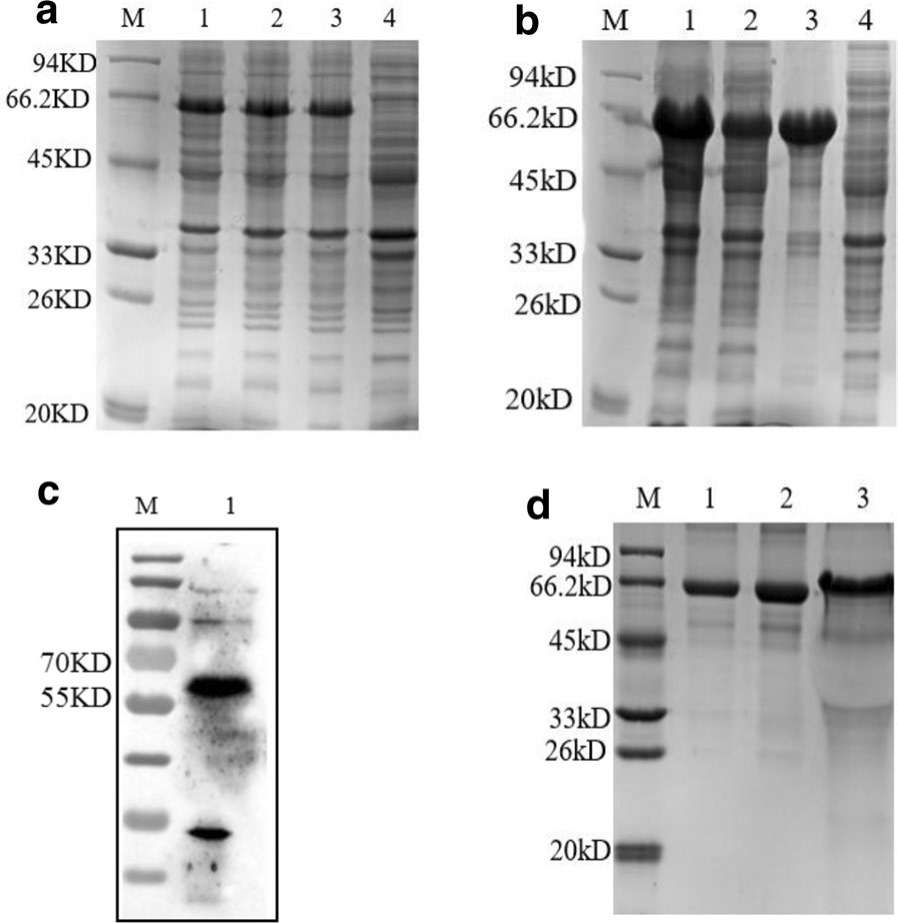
SDS-PAGE and western blot analysis of the rTgMIC1 protein. a Expression of pET30a-MIC1/BL21 in small amounts. Lane M: protein marker; lane 1–3: mono-clone picked from conversion plates after induction with IPTG; lane 4: uninduced control. b Large amounts of expression detection. Lane M: protein marker; lane 1: proteins in pET30a-MIC1/BL21 after induction with IPTG; lane 2: supernatant of pET30a-MIC1/BL21 bacteria after ultrasonication; lane 3: precipitation of pET30a-MIC1/BL21 bacteria after ultrasonication; lane 4: uninduced control. c Western blot analysis of the rTgMIC1. Lane M: protein marker; lane 1: rTgMIC1 detected by HIS monoclonal antibodies. d The rTgMIC1 purified by Ni^2+^-affinity chromatography. Lane M: protein marker; lane 1: 0.5 mg/ml BSA; lane 2: 1.0 mg/ml BSA; lane 3: purified rTgMIC1 proteins
